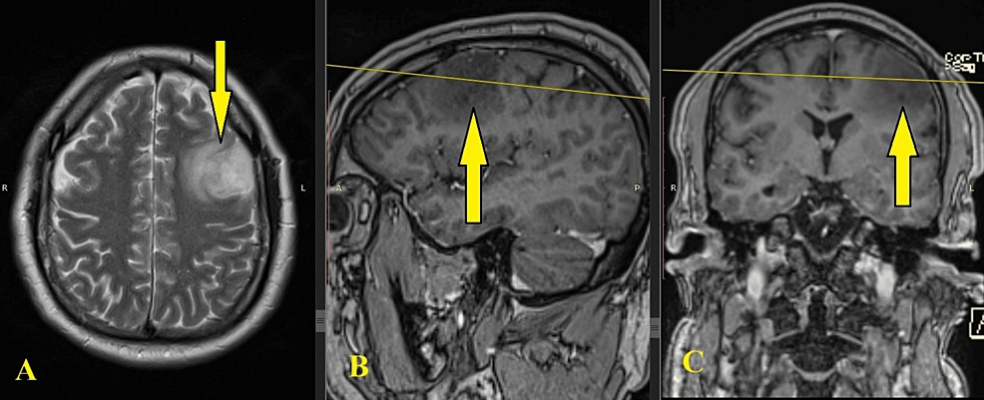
Preoperative MRI of left frontal glioma. (A) Axial plane; (B) sagittal plane; (C) coronal plane. Notice the tumor formation in the left frontal region of the cerebrum.
